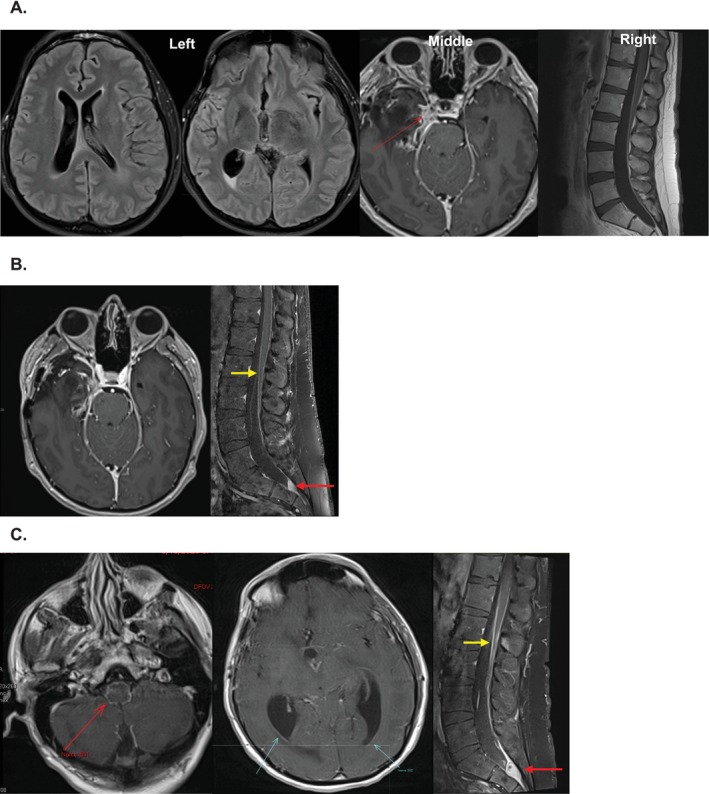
(A) Pre‐treatment MRI: Left: axial T2‐FLAIR images demonstrating enlarged lateral and third ventricles consistent with hydrocephalus. Periventricular edema notable surrounding the right occipital horn. Middle: axial post contrast image with nodular leptomeningeal enhancement in the right parasellar region (arrow). Right: Sagittal post contrast T1‐weighted images of lumbar spine with peripheral location of nerve roots and mild nerve root enhancement of the cauda equina nerve roots consistent with arachnoiditis. (B) Post‐treatment MRI: Left—at 4 months treatment: Follow‐up axial post contrast demonstrating stable nodular enhancement in the right parasellar region. Right—axial post‐contrast T1‐weighted image of the lumbar spine demonstrating linear leptomeningeal enhancement (yellow arrow) and a thecal sac metastasis (red arrow). (C) MRI showing progression at 7 months of treatment: Left and middle—Axial post contrast images demonstrating leptomeningeal enhancement surrounding the brainstem and ependymal enhancement of the bilateral occipital horns. Right—Sagittal post contrast images of the lumbar spine demonstrating progressive leptomeningeal enhancement of the lower thoracic spinal cord (yellow arrow), the cauda equina, and nodular intradural disease at the sacrum (red arrow).
